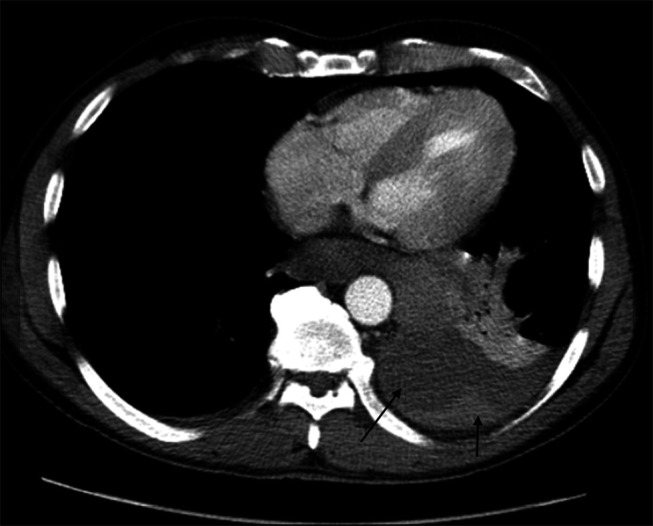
Computed tomography chest with contrast was performed which showed wall thickening of the mid-distal esophagus and gastric cardia with a large volume surrounding high-density material, favoring hematoma/hemorrhage, with a small focus of active contrast extravasation/bleeding medial to gastric cardia. Moderate left hemothorax is noted (black arrows).
